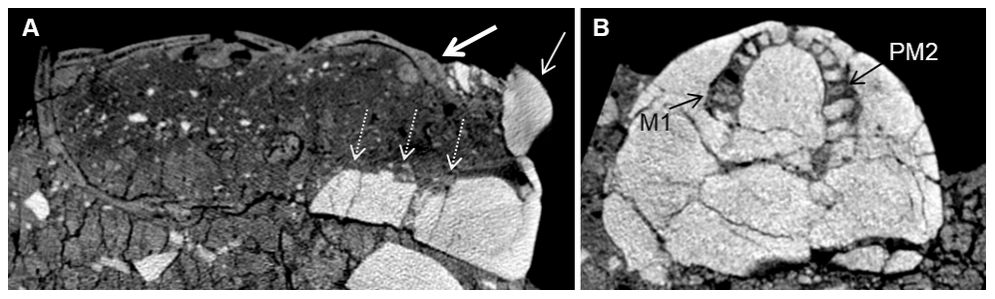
CT scan of Homo 2. In the sagittal section (A), note that the superior part of the plastered nose (thin arrow) ends at the level of the supraorbital ridge (thick arrow). There is therefore a discrepancy between the mask's features and the correct anatomical position. Note also the thick layer of plaster (dashed arrows) at the base of the skull, instead of the missing mandible. In axial section at the level of the maxilla (B), note that the cervical part of the dental sockets for most teeth are filled with plaster. The roots of the left first molar (M1) and the right first premolar (PM2) are present.
